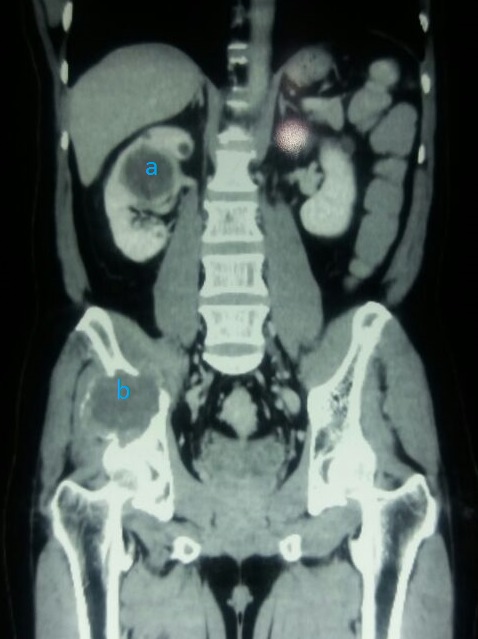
CT scan showing both (A) right renal and (B) pelvic tumours
